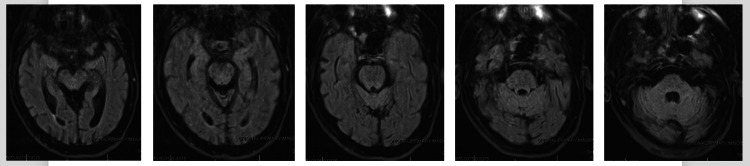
Brain MRI FLAIR The patient's brain MRI did not show abnormalities.
